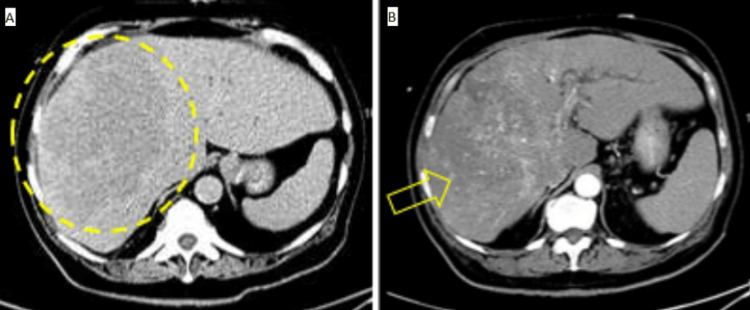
CT of the abdomen and pelvis with (A) and without (B) intravenous contrast demonstrating the hypervascular mass in the right hepatic lobe.
